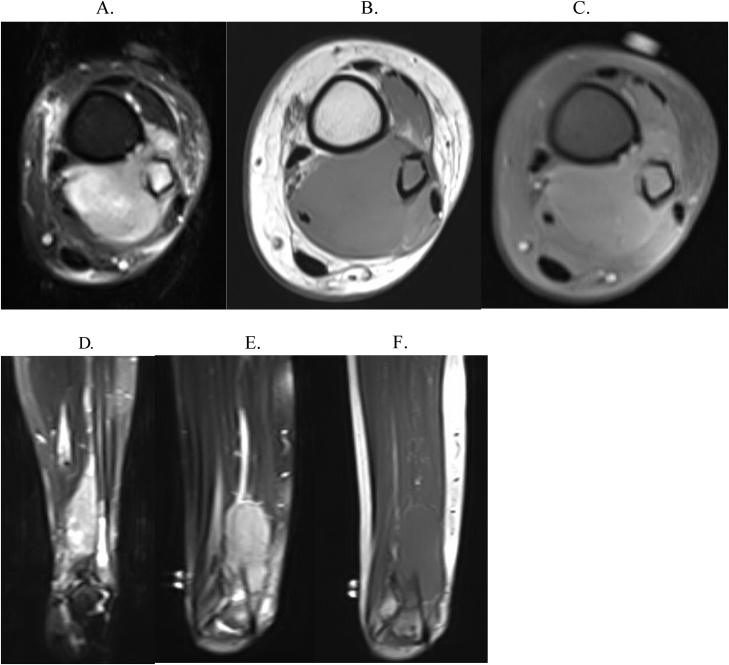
MRI of the left tibia/fibula of patient #2 A) Axial STIR sequence B) Axial TSE T1 C) Axial TSE T1 FS. D) Coronal STIR. E) Sagittal FSE T2 FS. F) Sagittal T1.
